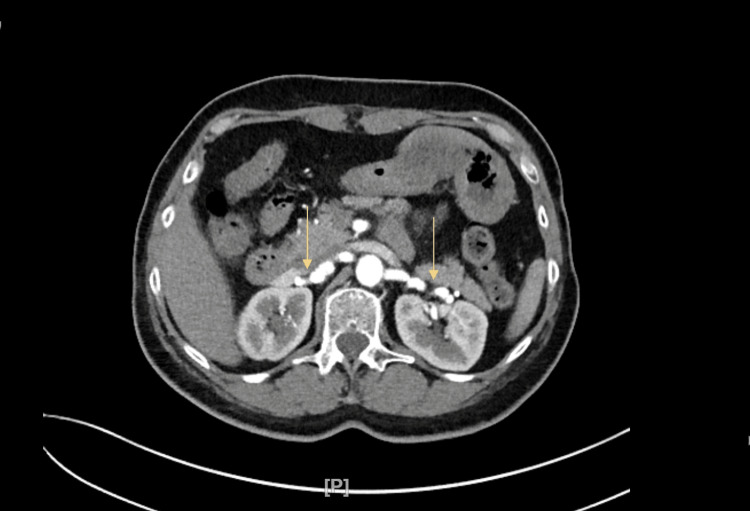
CT angiogram of the abdomen and pelvis with IV contrast The image shows the narrowing of the bilateral renal arteries, appearing as a string of beads CT: computed tomography
